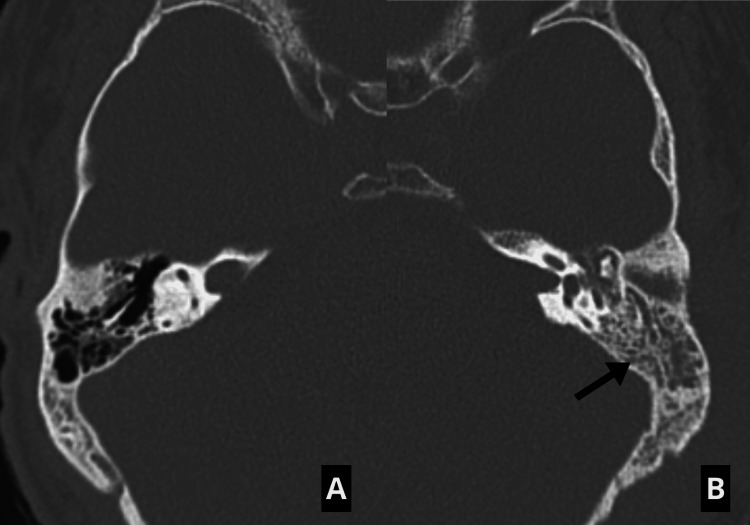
Computed tomography scan of the temporal bone (axial view) A: The right middle ear cavity and mastoid are normally aerated, with intact ossicles. B: The left side shows inflammatory material dispersed throughout the mastoid and much of the tympanic cavity (arrow), along with enlargement and mild erosion at the superior limit of the external auditory canal.
